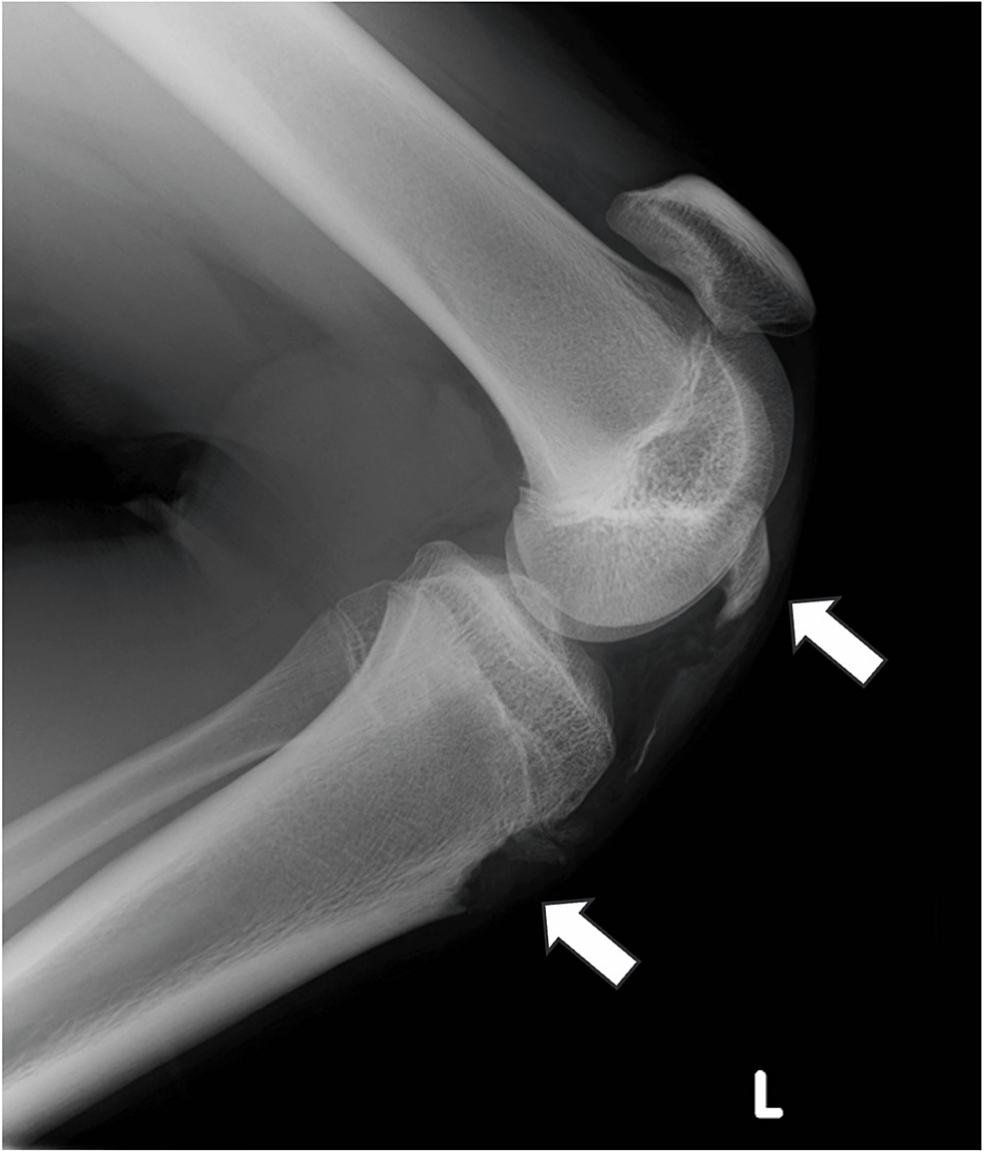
Preoperative radiograph shows Ogden type ⅠB tibial tubercle fracture.
